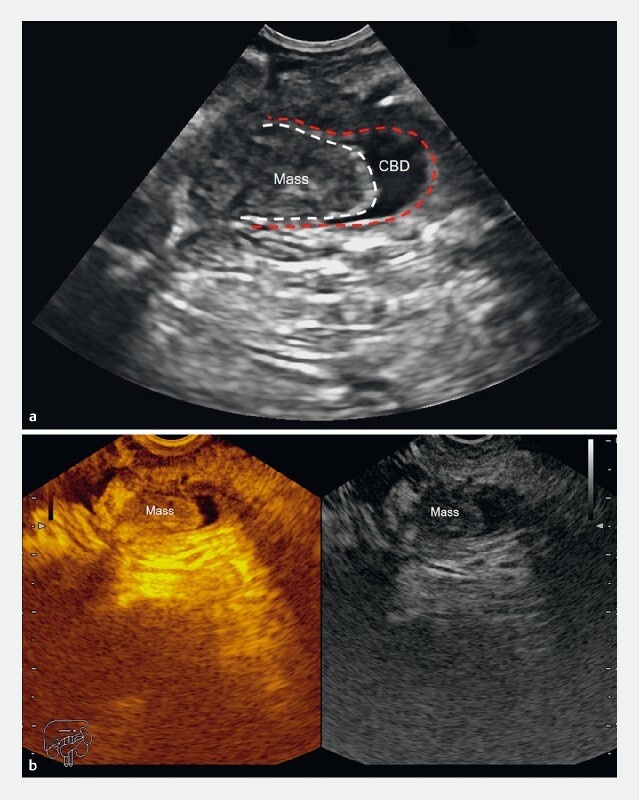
Endoscopic ultrasound images.
